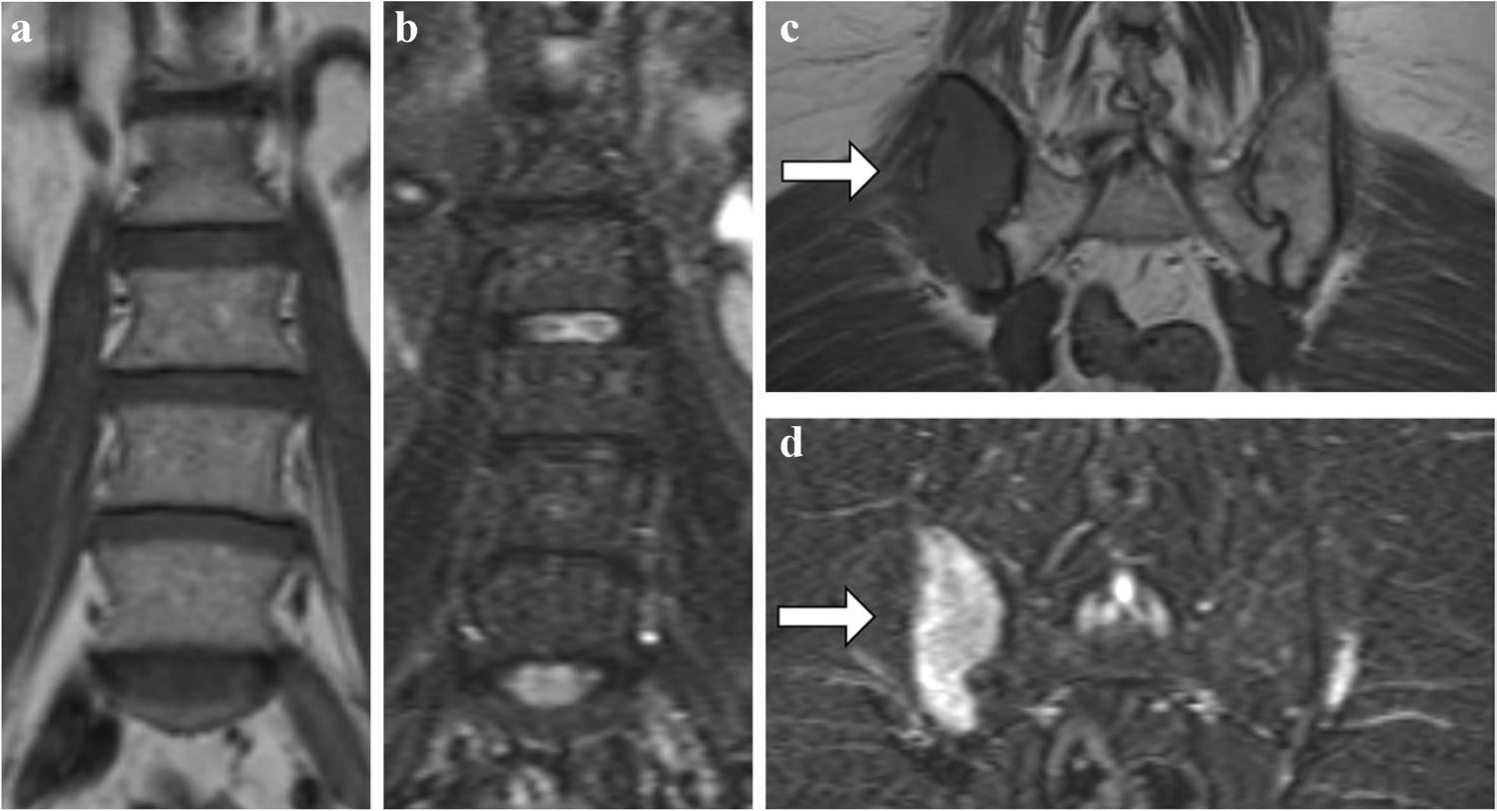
a–d Case 3. A 60-year-old female with multiple myeloma with 5% PCI after biopsy of mostly unaffected bone marrow in the posterior iliac crest contralateral to a large focal lesion. Coronal T1tse (a, c) and STIR (b, d) MR images show no diffuse infiltration in spine (a, b) or the left pelvis (c, d). Wb-MRI revealed a 6.8 cm by 3.0 cm large focal lesion at the right posterior iliac crest (c, d, white arrows), which would have led to a significantly higher PCI assessment if the right side instead of the left side would have been chosen randomly for biopsy
